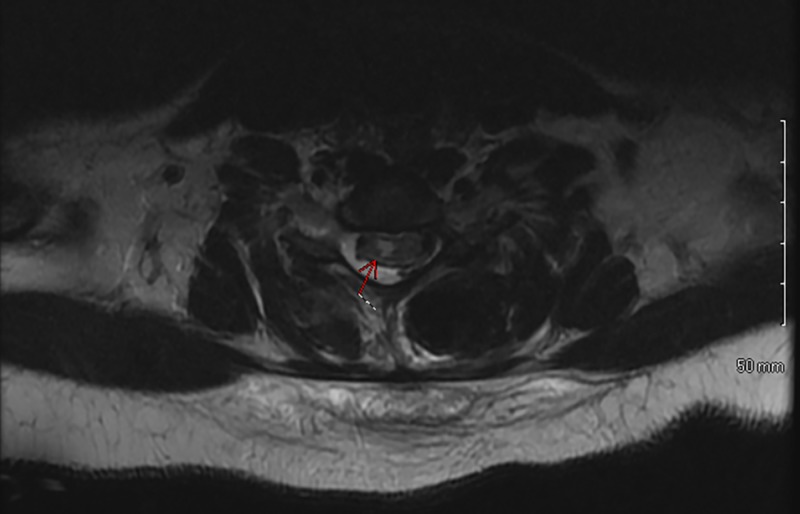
Postoperative axial MRI demonstrating acute myelomalacia changes of the spinal cord MRI: magnetic resonance imaging
